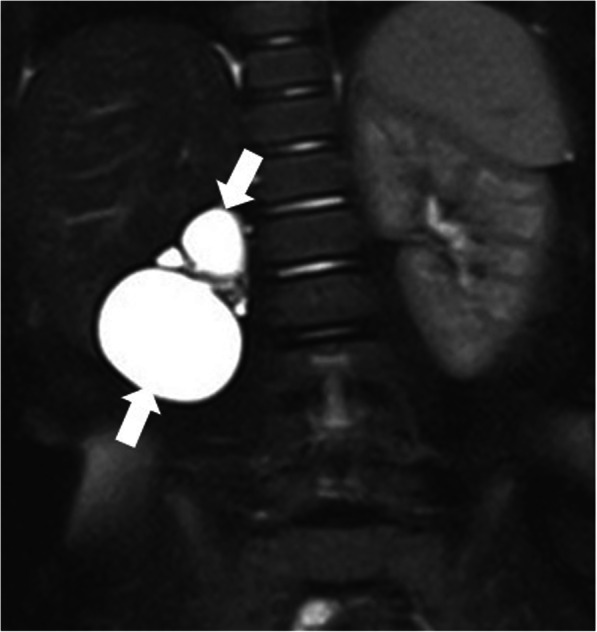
A 12-day-old newborn with prenatally diagnosed right kidney cysts underwent an abdominal MRI examination. Coronal plane T2W MR image shows multiple cysts completely replacing the right kidney parenchyma (arrows). There was no discernible renal parenchyma aside from the cysts. The left kidney was normal
